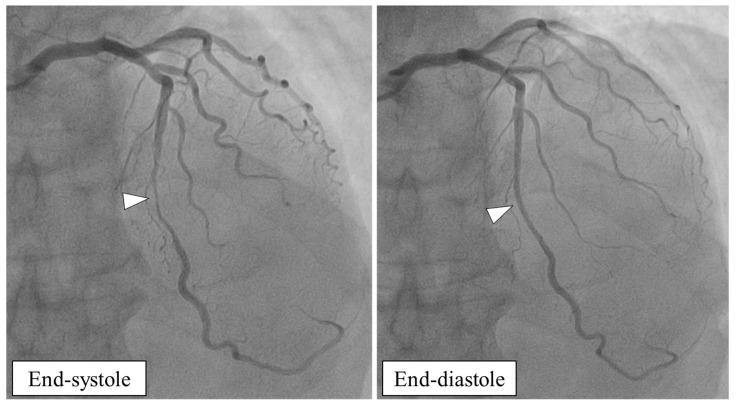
Invasive coronary angiography of a patient with isolated myocardial bridging (MB: arrow head) in the medial segment of the left anterior descending coronary artery (LAD). Note systolic compression of the intramyocardial LAD segment (“milking effect”) with reduced vessel luminal diameter at end-diastole. MB-patient with inducible myocardial ischemia on stress echocardiography and diastolic fractional flow reserve (d-FFR) ≤ 0.76.
